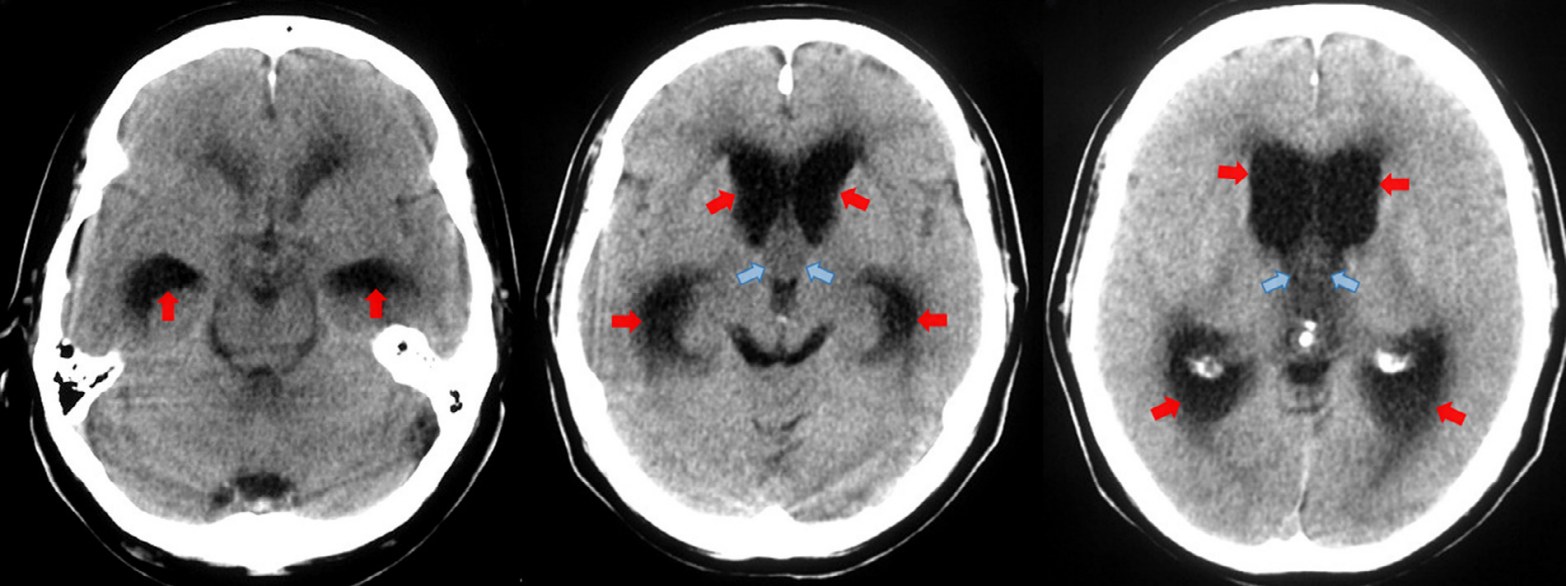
Axial CT images without contrast demonstrate a well-defined homogeneous hypodense nodular lesion (*blue arrows*) located between the foramina of Monro and the anterior portion of the third ventricle, causing symmetrical expansion of the lateral ventricles (*red arrows*).
